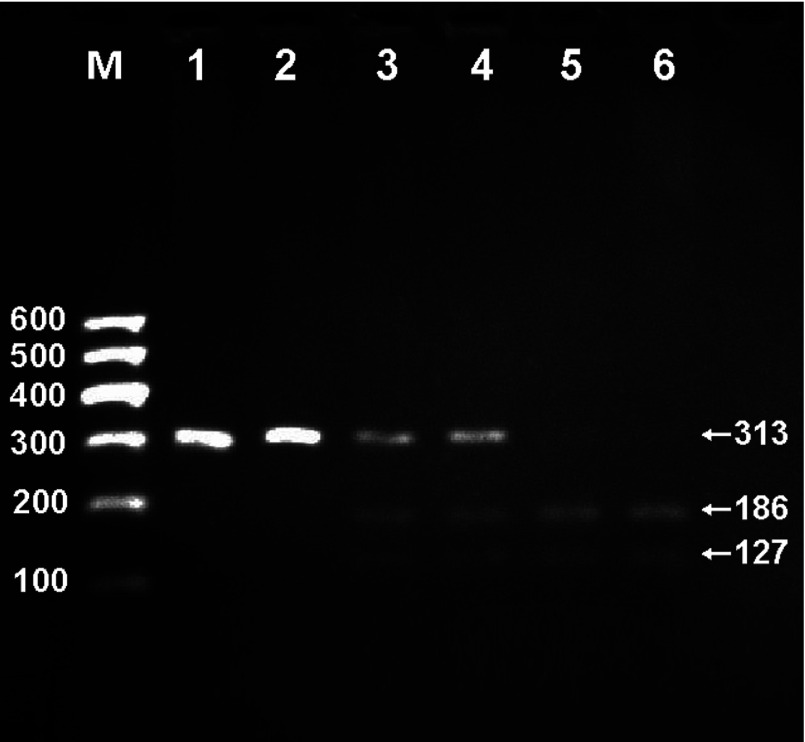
Genotyping of the TMEM57 rs873308 SNP Lane M is the 100 bp Marker ladder; lanes 1 and 2, CC genotype (313-bp); lanes 3 and 4, CT genotype (313-, 186- and 127-bp); and lanes 5 and 6, TT genotype (186- and 127-bp).
